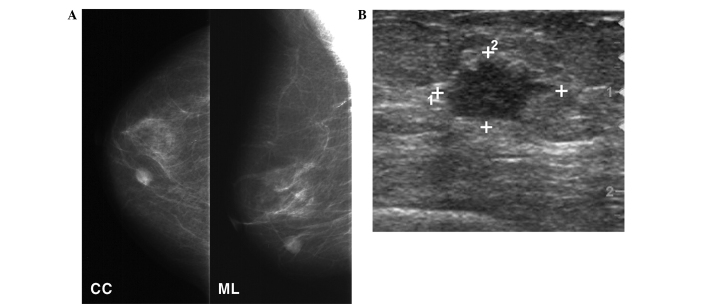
Secretory breast carcinoma in Case 1. (A) Mammography showing a round, high-density mass in the lower inner quadrant of the right breast. (B) Ultrasonography showing a 1.3-cm, speculated, irregularly shaped hypoechoic mass in the breast.
